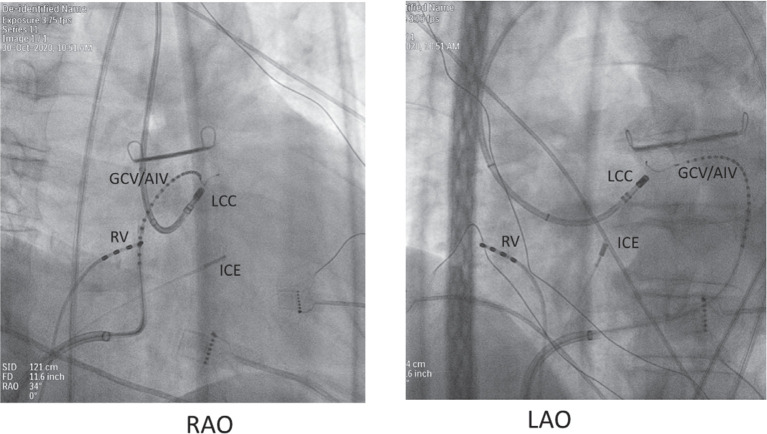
Right anterior oblique (RAO, left panel) and left anterior oblique (LAO, right panel) fluoroscopic views in a patient undergoing ventricular tachycardia ablation. A 20-electrode microcatheter is advanced via the CS to the GCV/AIV region. An ablation catheter is shown with its tip in the left coronary cusp, demonstrating the proximity of the left coronary cusp to the distal GCV. ICE: intracardiac echocardiography catheter; RV: right ventricular catheter.
